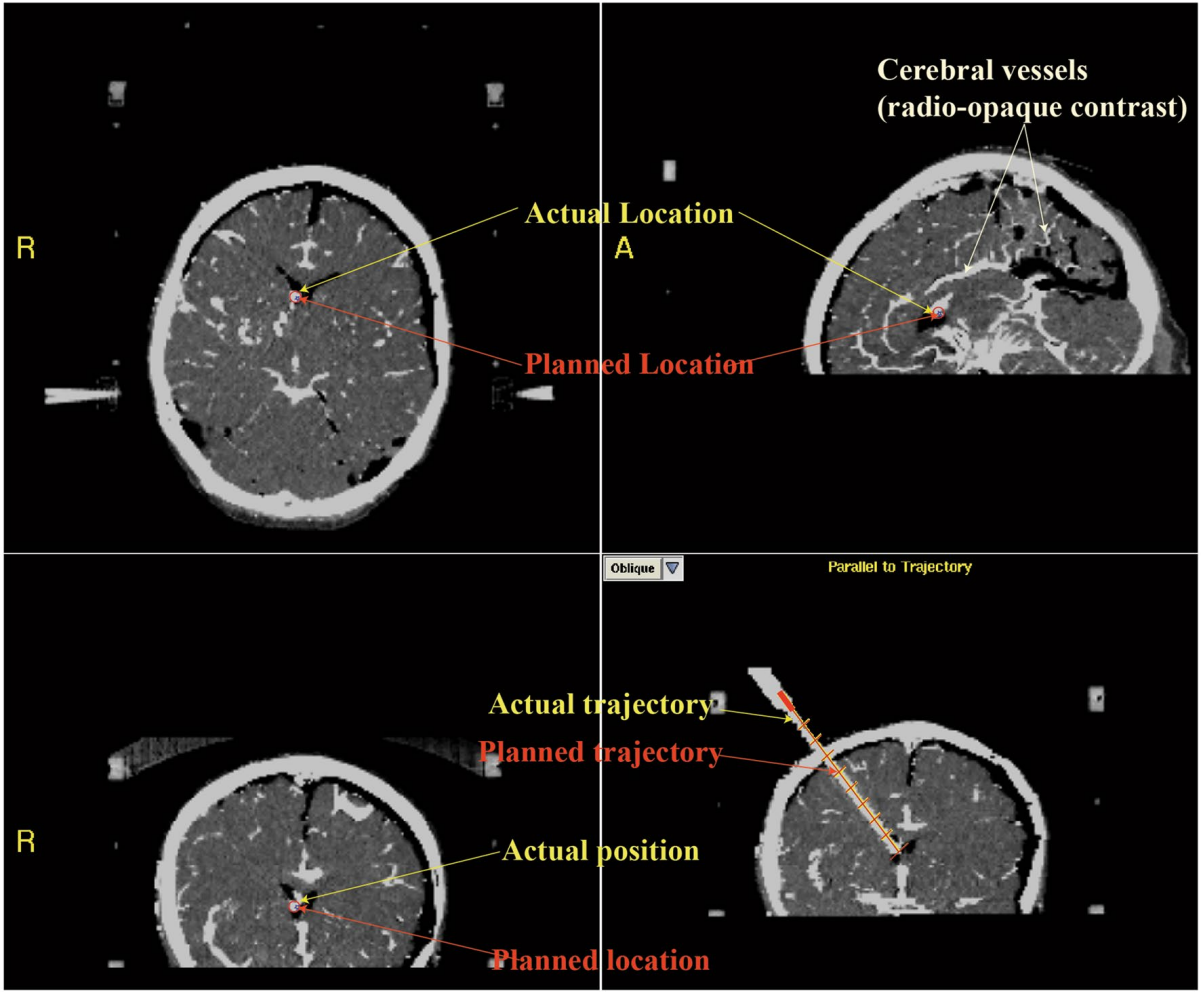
CT images showing robust targeting accuracy in a representative pre-clinical experiment (COMPASS software). The actual location of catheter tip (red circle) is seen to be overlying the planned location (blue dot). The bottom right panel shows the actual catheter trajectory (white glow) with superimposed planned trajectory (red line). Cerebral vasculature was avoided while placing the catheter.
